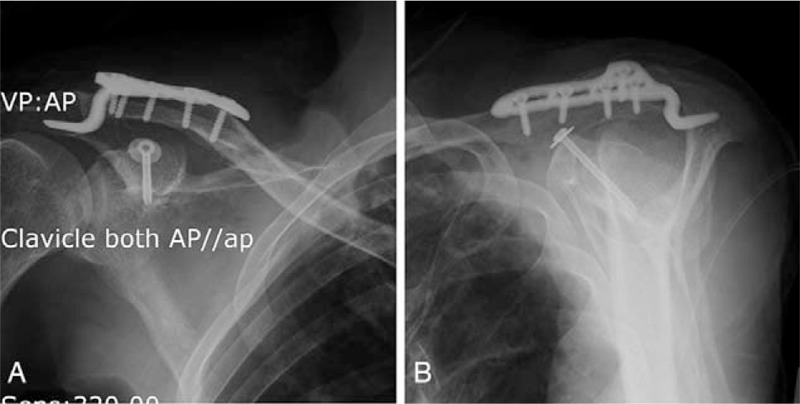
AC joint was fixed by LCP clavicle hook plate; the CP was fixed with a 3.5 mm diameter cannulated screw. Immediate postoperative anteroposterior X-ray view (A), immediate postoperative outlet X-ray view (B).
